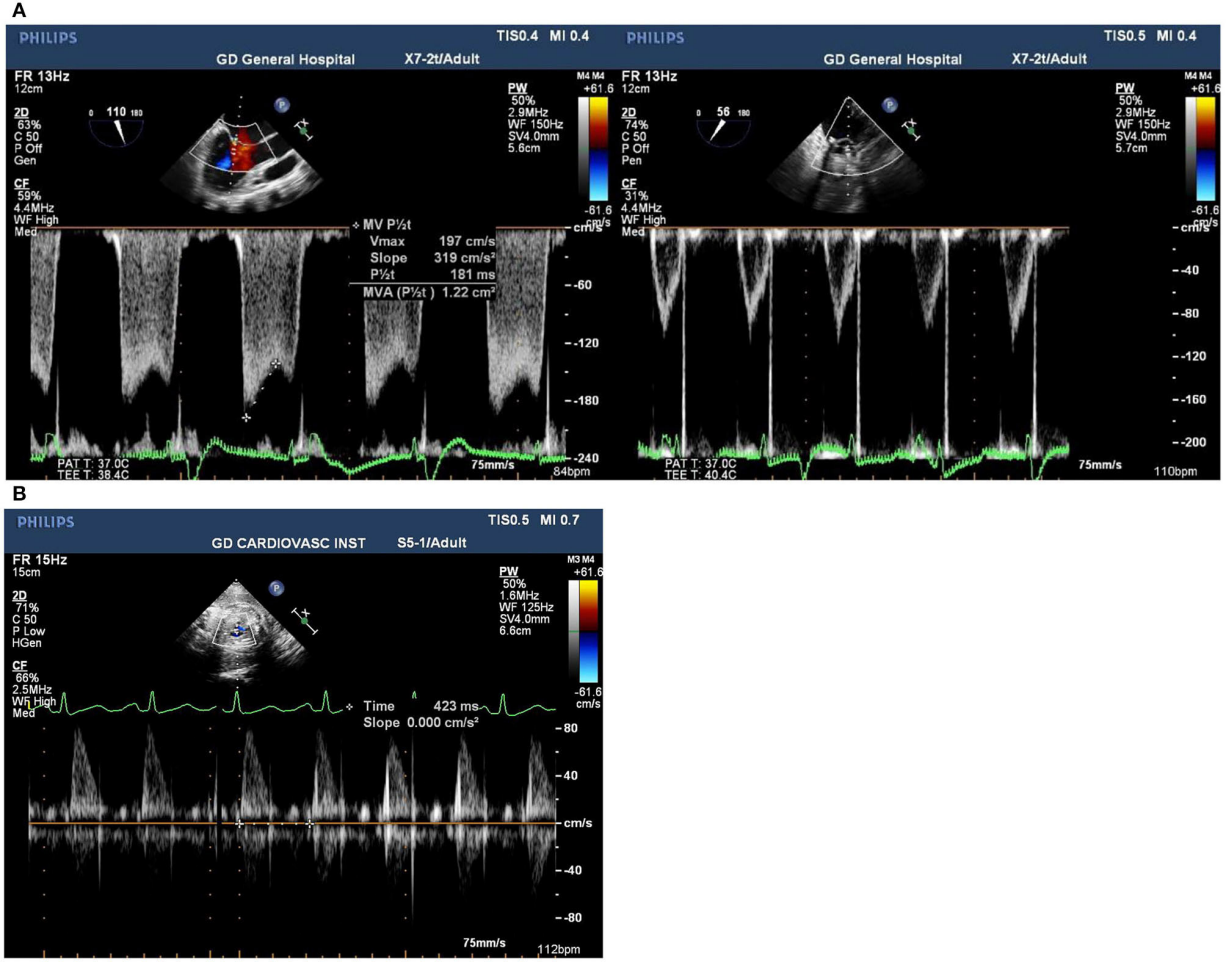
(A) Pre and post-surgical mitral valve view of transesophageal echocardiogram images of mitral valve stenosis in Case 1. (B) Postoperative transesophageal echocardiogram images of Doppler of fetal blood flow in Case 3 presenting the fetal heart rate at 141 bpm.
